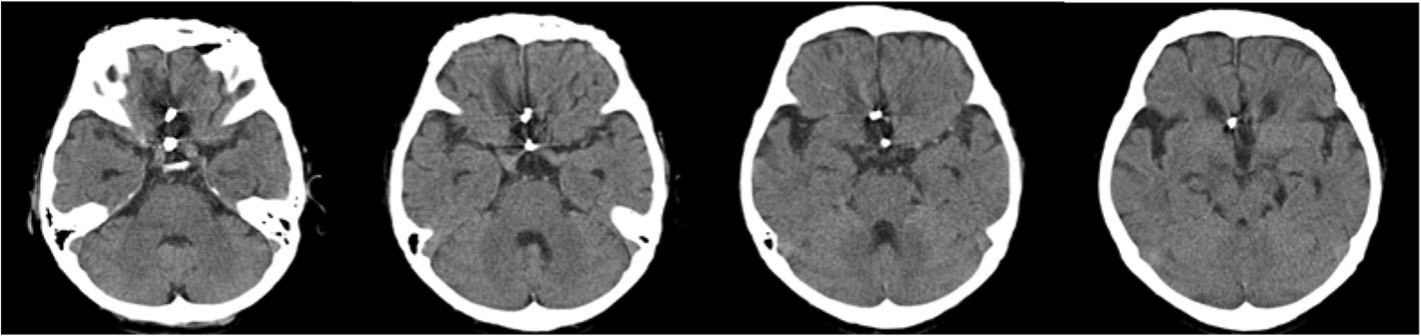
Computed tomography imaging of the patient’s head, showing a low-density area in the bilateral orbitofrontal cortex subgenual cingulate area and surgical clippings for the rupture of the anterior-communicating artery aneurysm and to prevent rupture of the right anterior cerebral artery aneurysm
